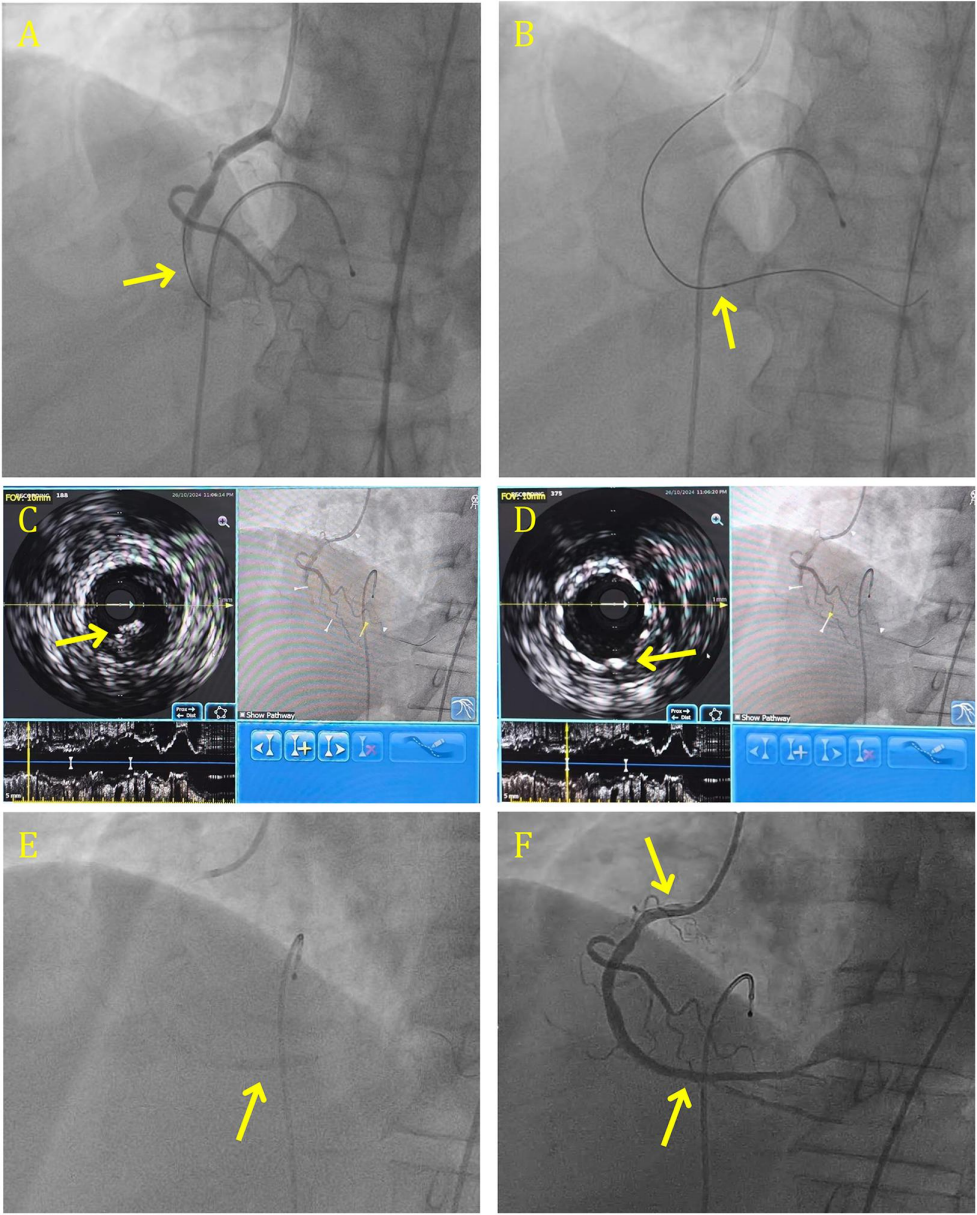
Large thrombus burden angioplasty done aided by ELCA. (A) Pre-PCI CAG shows mid RCA 100% occluded with grade 6 clot & TPI. (B) CAG shows the successful crossing of lesion with ELCA (Pulses 40–80 in 3 rows). (C) IVUS post ELCA still visible clot. (D) IVUS post stent fully expanded. (E) CAG shows the persistent clot in the distal PLV branch, prompting direct stenting with a 3.0 × 20 mm DES in the PLV. (F) Good final result with TIMI 3 flow filling distal RCA, PLV & PDA. PCI, percutaneous coronary angiography; CAG, coronary angiography; RCA, right coronary artery; TPI, temporary pacemaker implantation; ELCA, excimer laser coronary angioplasty; IVUS, intravascular ultrasound; PLV, posterior left ventricular; PDA, patient ductus arteriosus.
